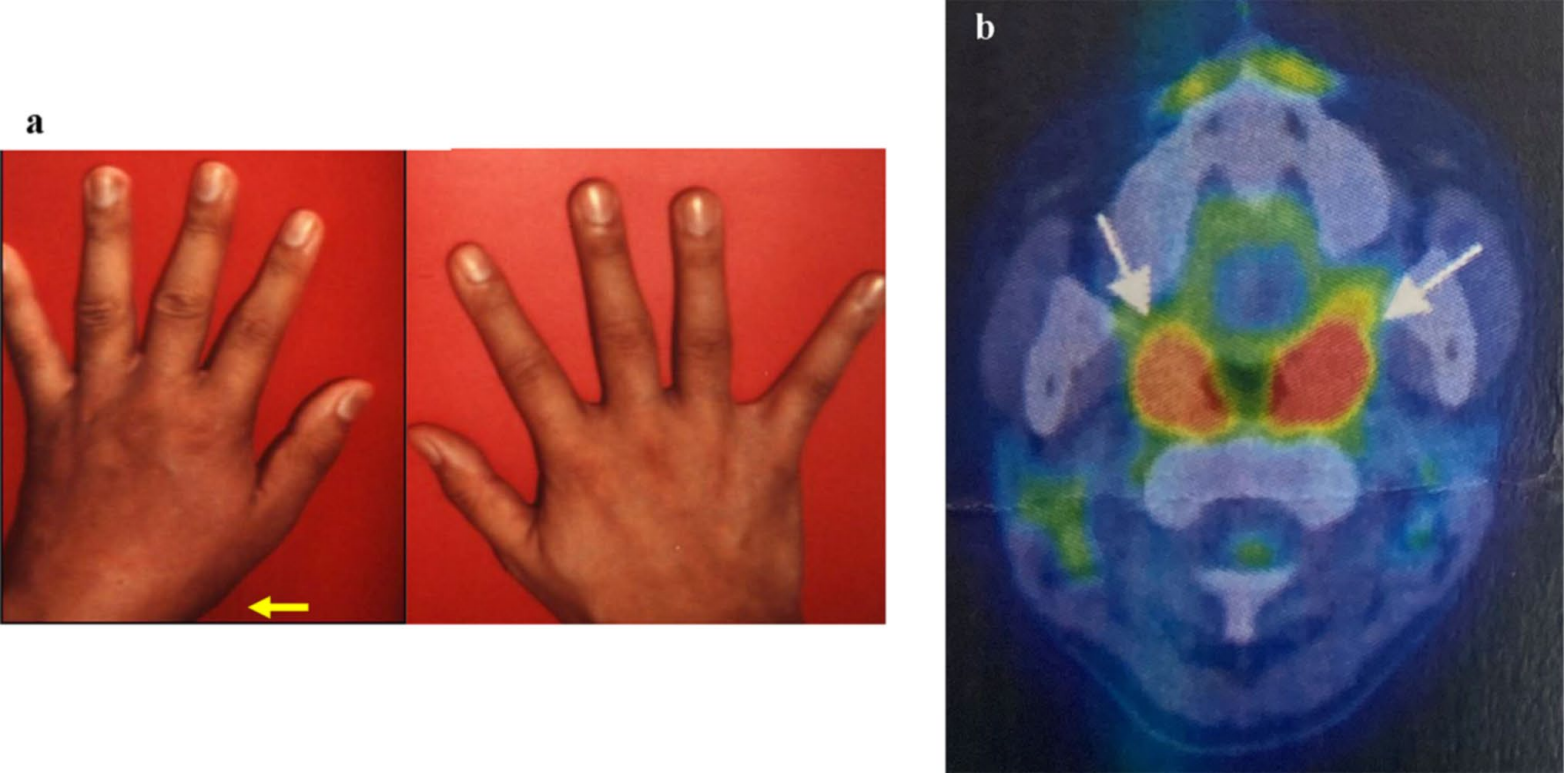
Arthritis of left wrist and image of patients with tonsillitis-related arthritis. Arthritis of either side of the wrist and tonsillitis recurred for 10 years. The left wrist inflammation improved 1 week after antibiotic therapy. *Pseudomonas aeruginosa* was the only bacteria found in a culture of the resected tonsils (a) [52]. Positron emission tomography/computed tomography shows the inflamed palatine tonsils of a 38-year-old woman with recurrent tonsillitis and arthritis (b)
